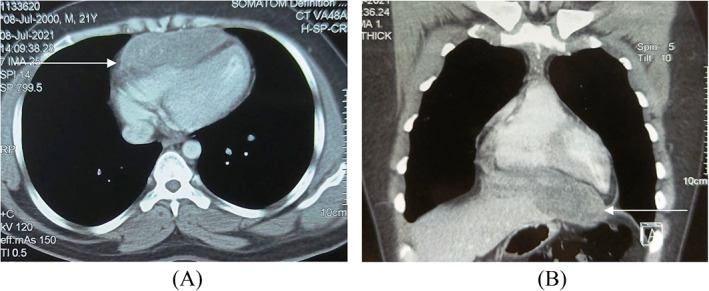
Contrast enhanced CT of chest (A) axial and (B) coronal images showing a heterogeneously hyperdense mass in relation to inferior surface of heart and diaphragm (arrow indicates the mass).
